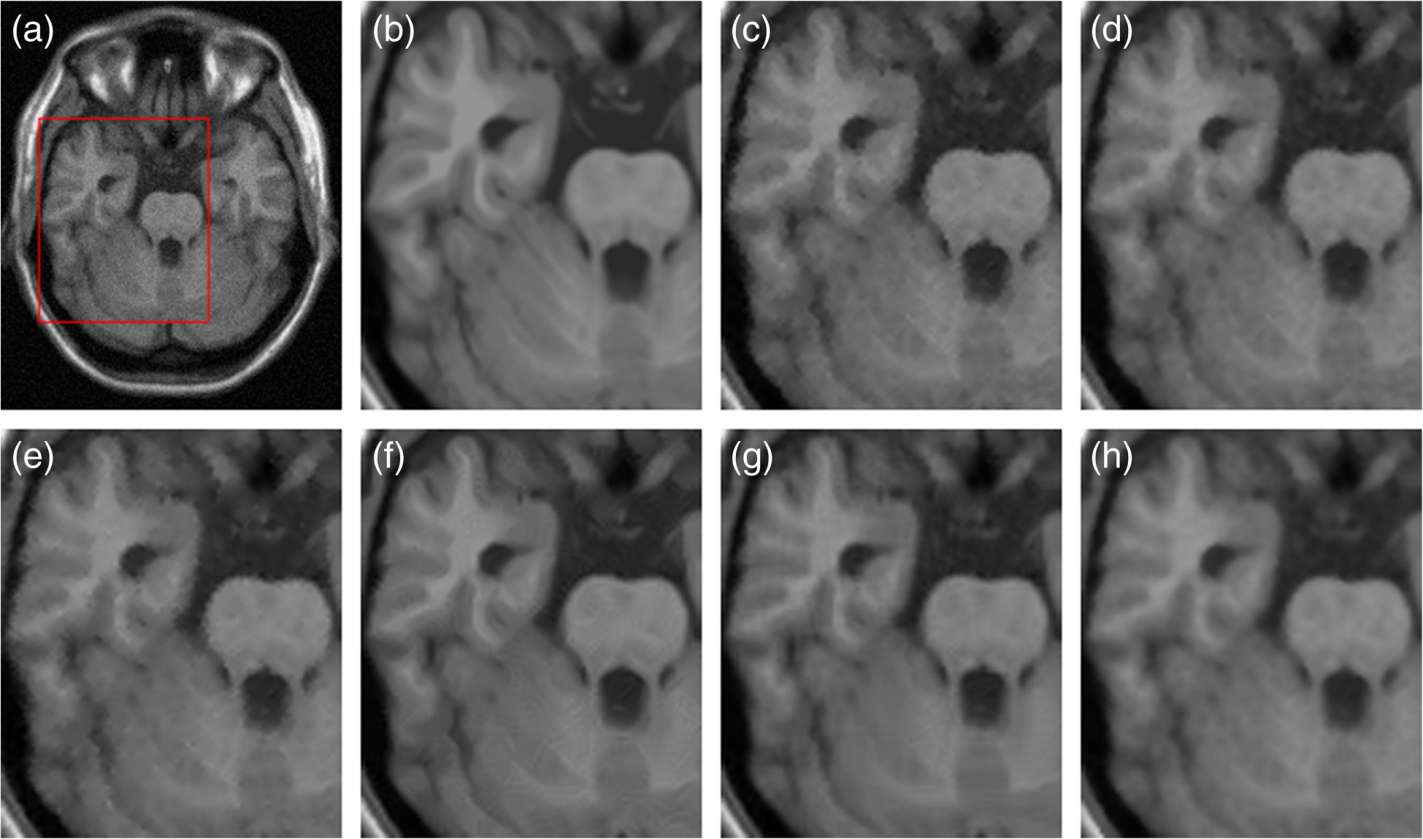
Comparison of visual restoration results on slice 10 with 5% noise in the 5 mm MS dataset. a Noisy MR image. b Intact image in magnified view. c IBF with PSNR = 32.49 and SSIM = 0.8650. d ADF with PSNR = 33.61 and SSIM = 0.8684. e LMMSE with PSNR = 32.53 and SSIM = 0.8657. f NLM with PSNR = 32.53 and SSIM = 0.8800. g BM3D with PSNR = 32.49 and SSIM = 0.8997. h Proposed with PSNR = 34.32 and SSIM = 0.8803
